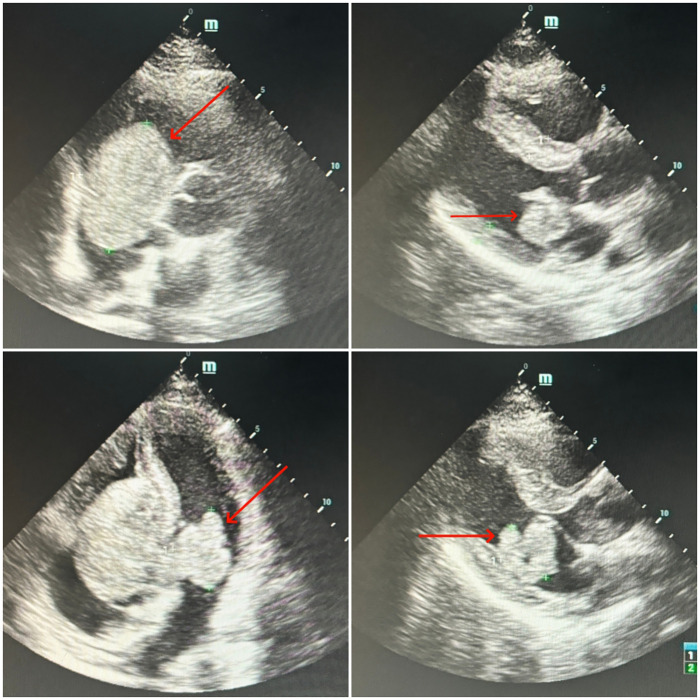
Echocardiography demonstrated giant hyperechoic masses occupying both the left and right cardiac cavities.
